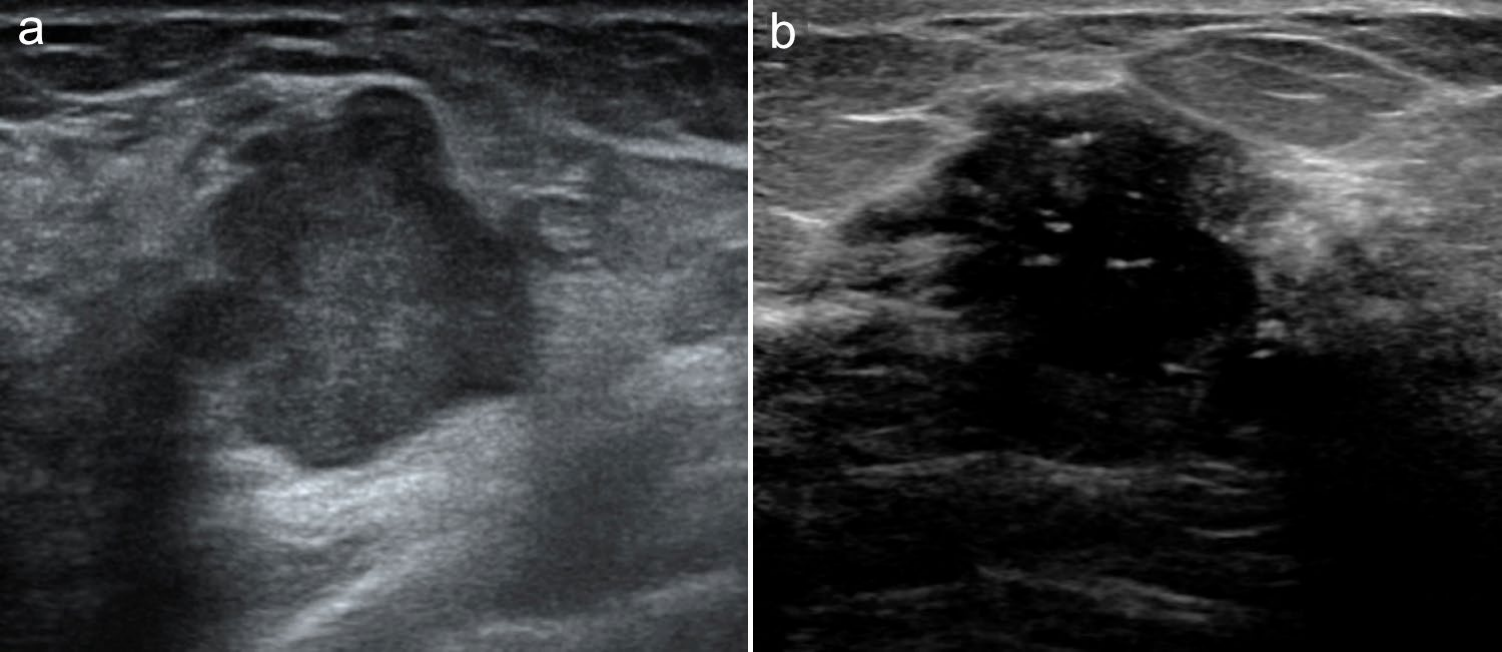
Pre-NACT ultrasound images of two patients with HER2-positive breast cancer who obtained different pathologic responses. a Breast ultrasound image of a pCR patient showing blurred nodal margin and posterior acoustic enhancement. b Breast ultrasound image of a non-pCR patient showing spiculated margin, internally scattered strong echogenic spots and posterior acoustic attenuation
